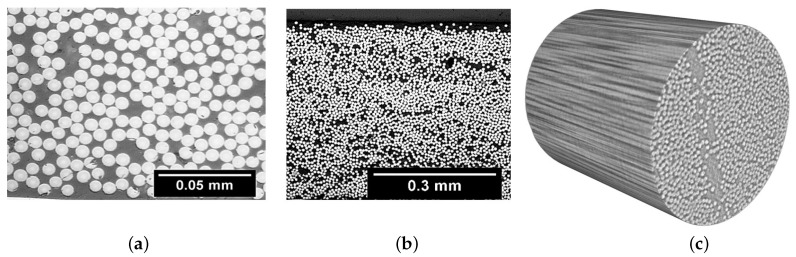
Inspection of AS4/PA12; (a) A high magnification. (b) A low magnification. (c) 3D CT image.
